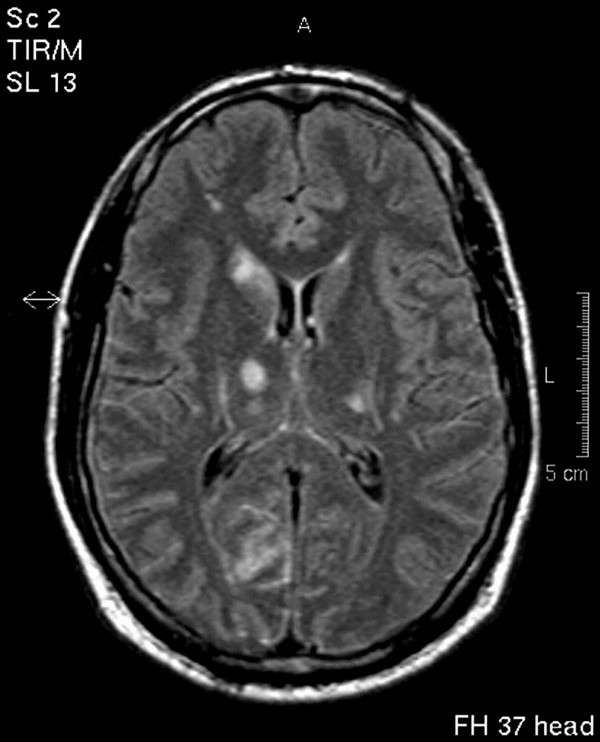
Cranial MRI (FLAIR) on the day of admission showing multiple lesions of the supratentorial grey and white matter.
